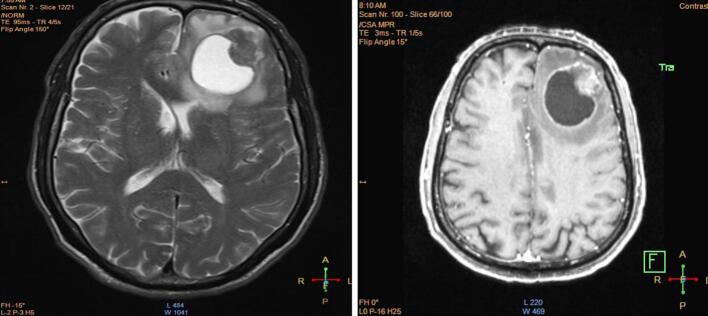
Magnetic resonance images of the brain demonstrating differences in T1- and T2-weighted contrast. Left image (T2-weighted): This axial slice was acquired with a turbo spin echo sequence using an echo time (TE) of 95 ms, repetition time (TR) of 4/5 s, and flip angle of 150°. T2-weighted images produce high signal intensity from fluid-containing structures such as edema due to the longer TE, making them optimal for visualizing pathology involving increased water content. Right image (T1-weighted post-gadolinium contrast): This coronal slice was obtained following intravenous administration of a gadolinium-based contrast agent using a spin echo sequence with a TE of 3 ms, TR of 1/5 s, and flip angle of 15°. In T1-weighted images, fat and tissues such as gray matter appear bright. Regions of blood-brain barrier disruption, as occurs with tumors, infections, or areas of inflammation, enhance following contrast administration due to leakage of gadolinium chelate into the extracellular space. This facilitates identification and characterization of lesion morphology and extent.
